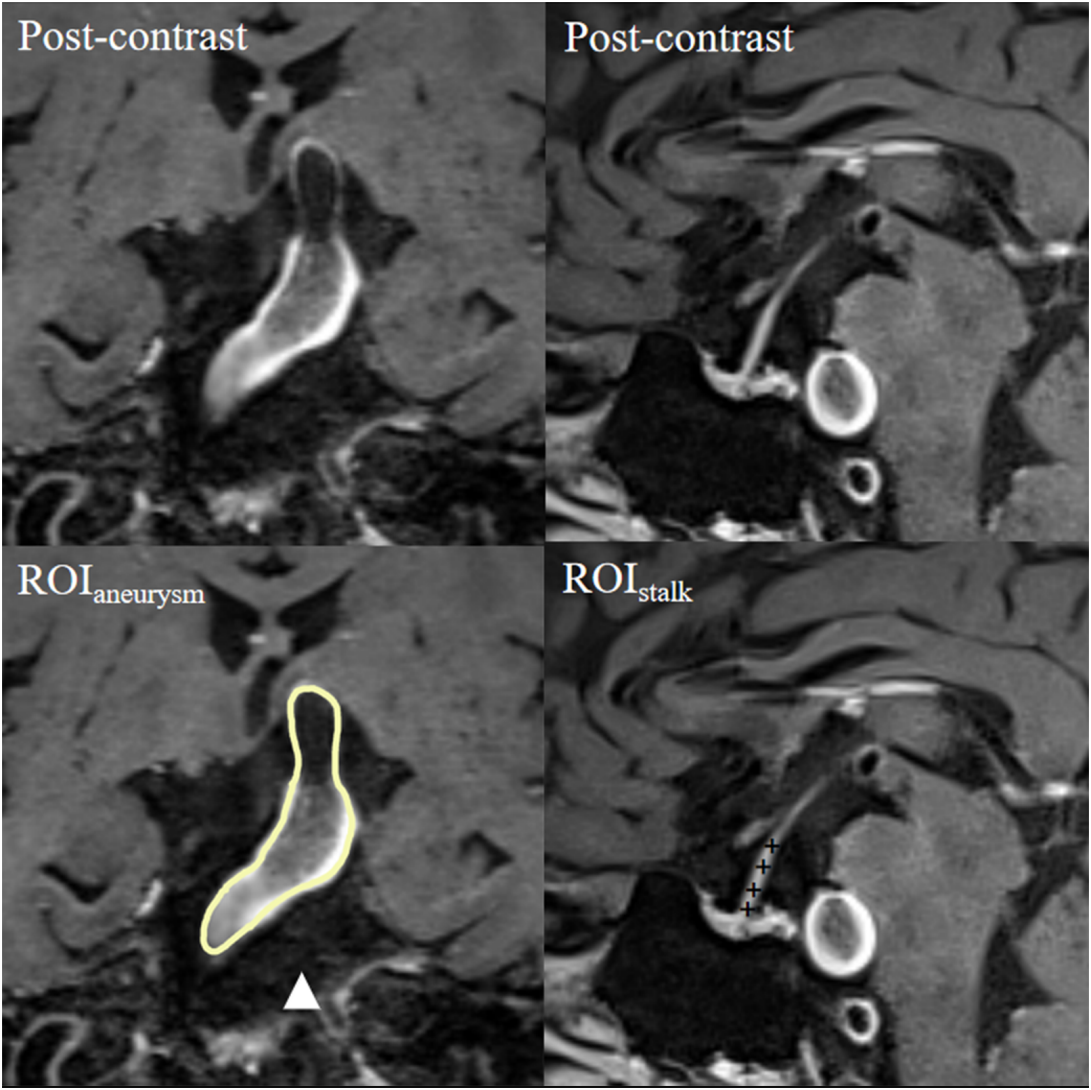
Representative case of aneurysmal wall enhancement assessment. The first row shows post-contrast T1-weighted images of a basilar fusiform aneurysm and a sagittal view of the pituitary stalk. The second row shows the ROIs of the aneurysm and pituitary stalk. The average signal intensity of the ROI of the aneurysm (ROI_aneurysm_) and the average signal intensity of four randomized points inside the ROI of the pituitary stalk (ROI_stalk_) were measured. CR_stalk_ was calculated as the contrast ratio of the signal intensity of ROI_aneurysm_ to ROI_stalk_. CR, contrast ratio; ROI, region of interest.
